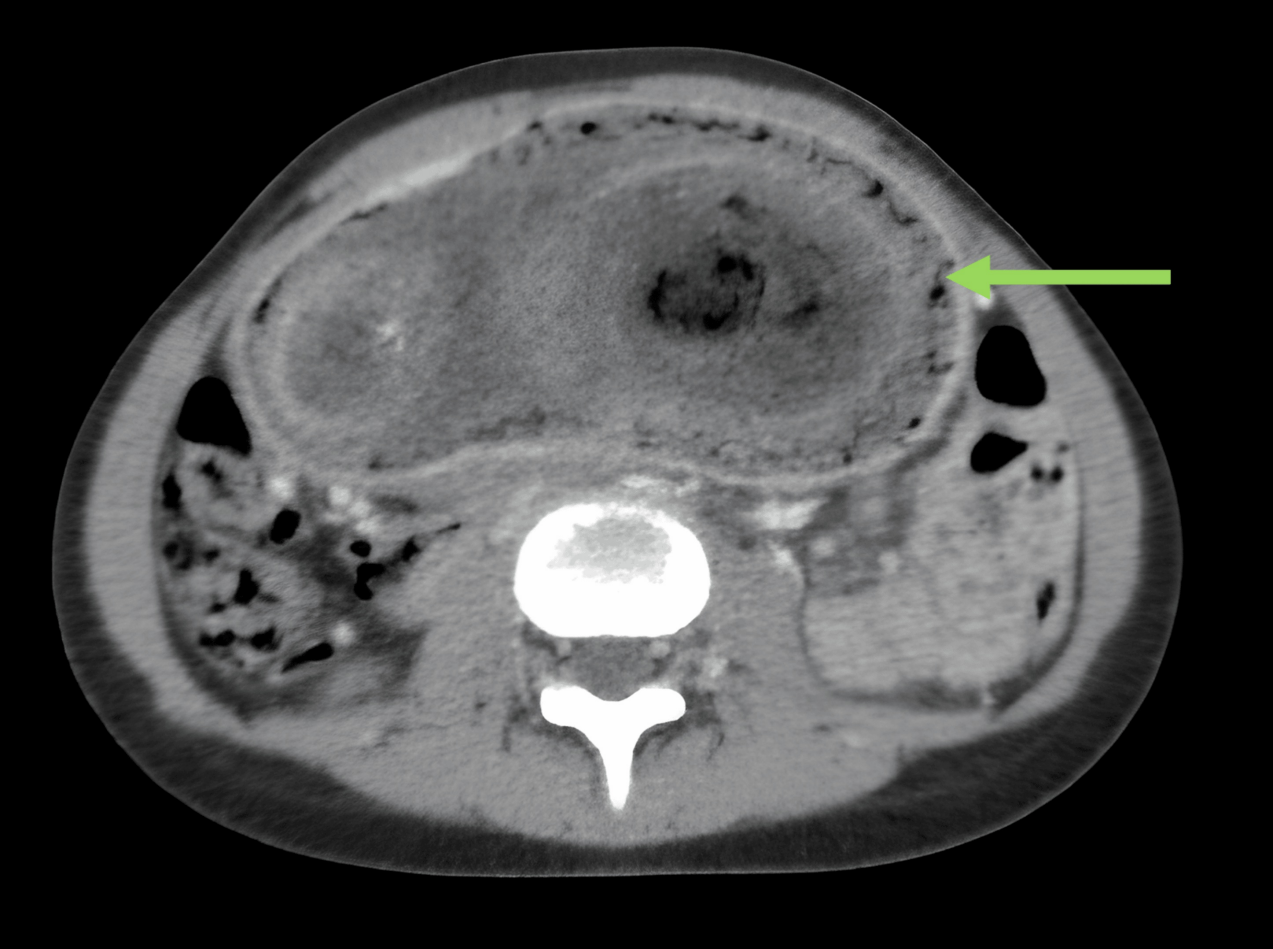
Abdominal CT ( transverse section) showing a thin wall enhanced after contrast injection (arrow).
